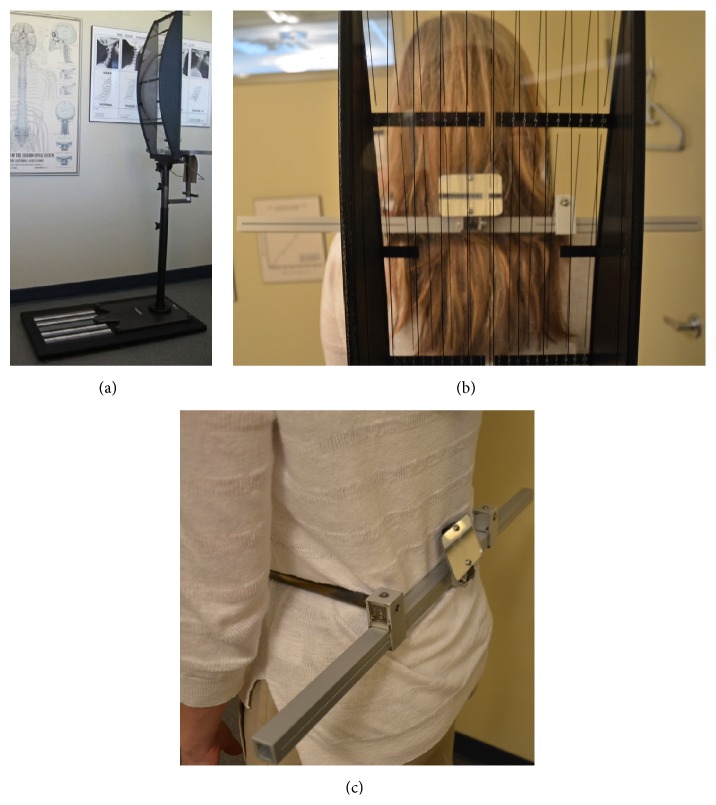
Gravity Stress Analyzer (GSA). (a) Device determines postural asymmetry as a further indicator of atlas misalignment. Positive findings in the SLC and GSA indicate need for NUCCA radiographic series. (b) Balanced patient with no postural asymmetry. (c) Hip calipers used to measure pelvis asymmetry.
